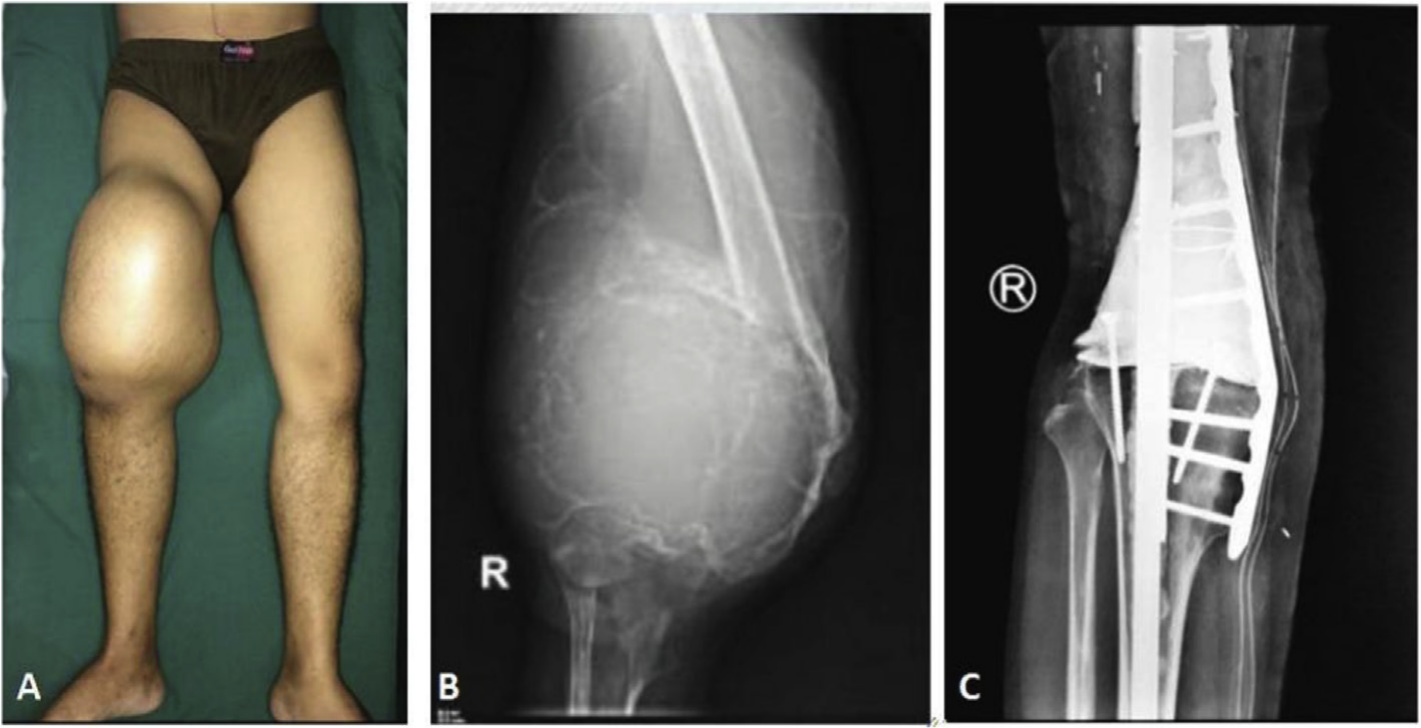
En bloc resection of the GCT at right distal femur and reconstruction with MAMC. A). Clinical picture of huge mass of the GCT at the right distal femur; B).Radiograph of the patient before surgery; C). Postoperative radiograph with MAMC
